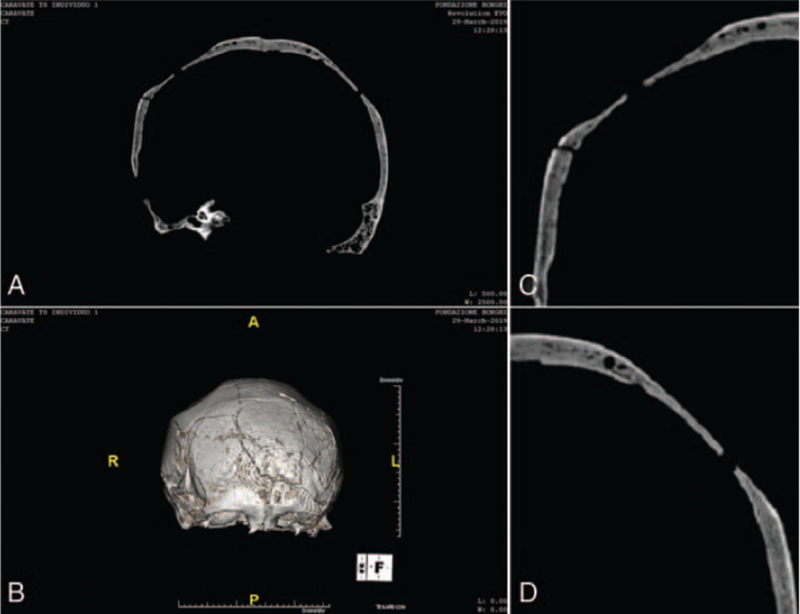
CT coronal vision, symmetrical depression of the parietals, (A) resorption of the external table and of the diploe, (B) 3d reconstruction of the skull, (C) left lateral view, (D) right lateral view. CT, computer tomography.
